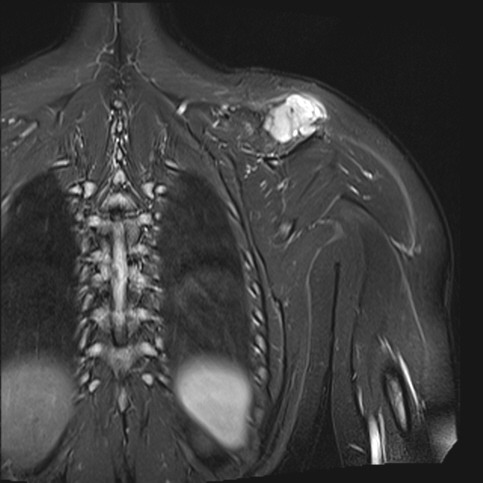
Figure 1:MRI revealed a soft tissue lobulated mass measuring 41 cm x 26 mm in the superior aspect of supraspinatus muscle.
